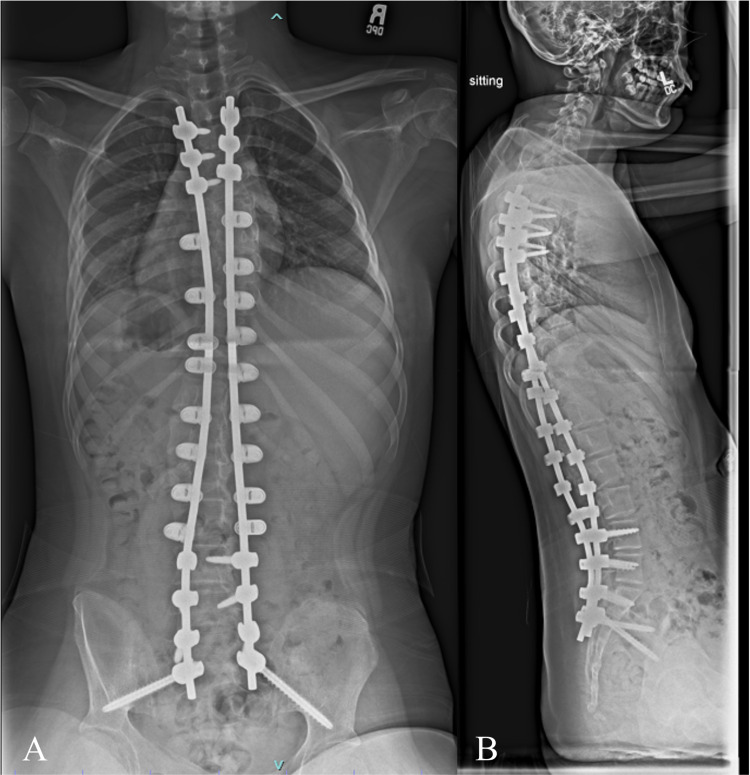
A) PA and B) lateral x-rays from one-year post-operative visit demonstrating fusion mass posteriorly, maintained deformity correction, and intact implants.
